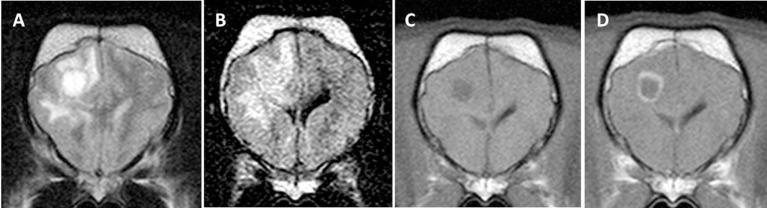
Transverse magnetic resonance images (0.5 T) of a French Bulldog (2 years, female) with necrotizing leukoencephalitis. T2 (A) and fluid-attenuated inversion recovery (FLAIR) (B) hyperintense round-shaped lesion accompanied by secondary white matter edema in the right frontal lobe. The T2 signal of the round center is partially suppressed on the FLAIR sequence indicating a liquid-like texture being consistent with necrosis. There is a ring-like contrast enhancement around the necrotic center after contrast injection [(C) T1-weighted native; (D) T1 weighted after contrast injection].
